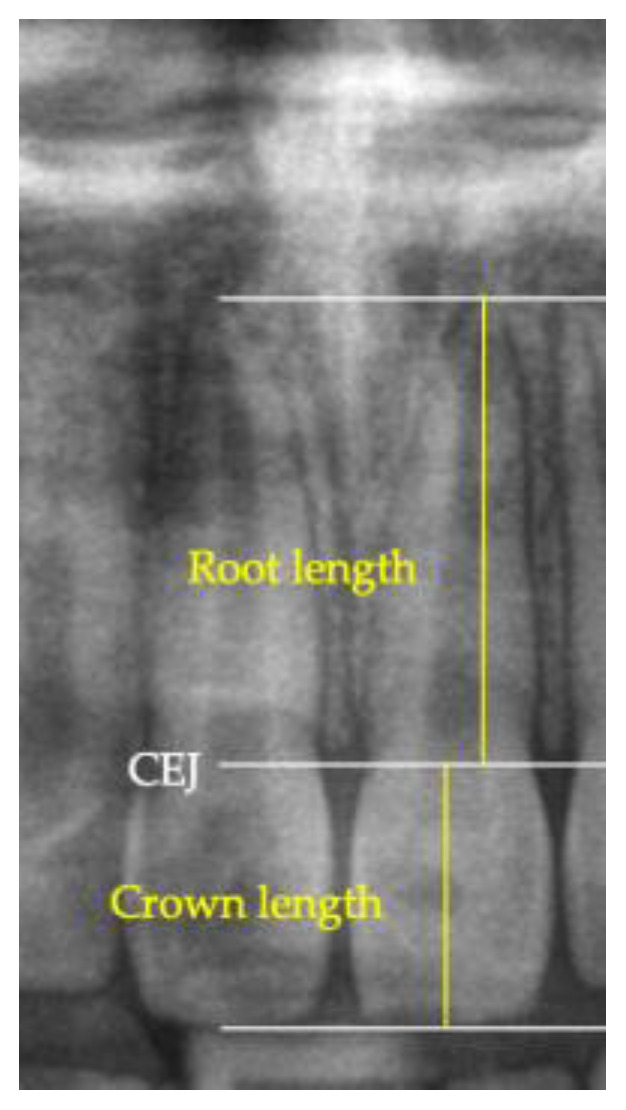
The measurement of crown and root length of tooth 21 on the panoramic radiograph.
